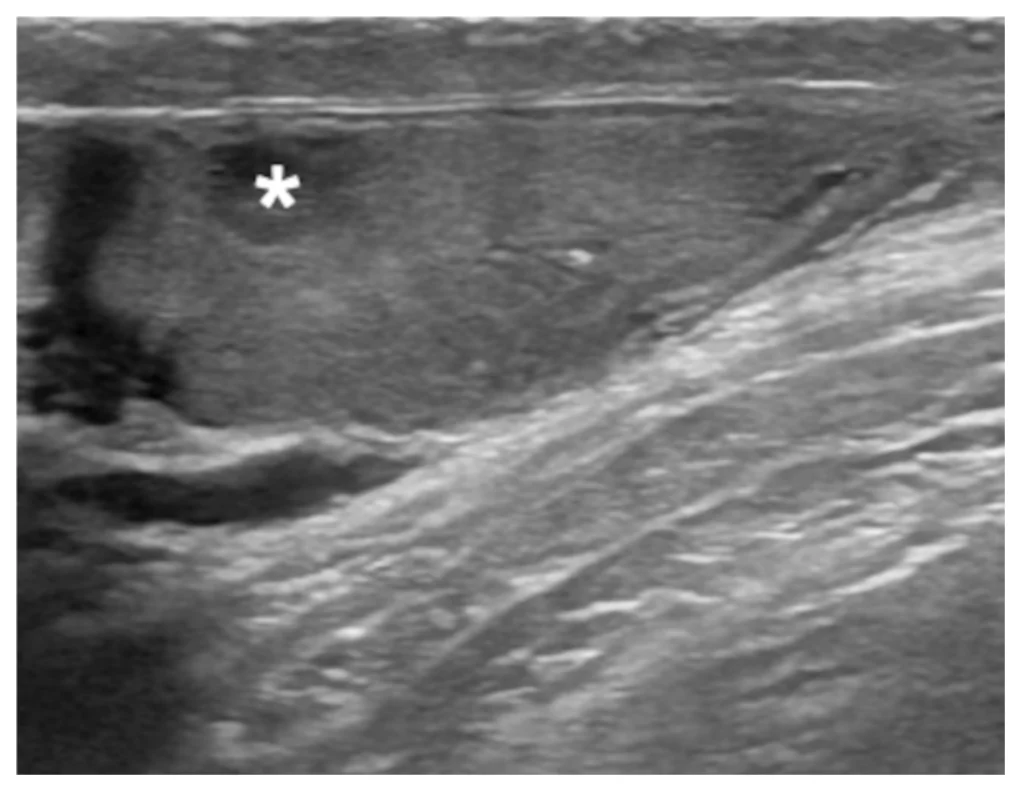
Small, non-palpable testicular lesion (asterisk) incidentally identified at ultrasound in a 45-year-old azoospermic man with bilateral testicular hypotrophy, investigated during fertility work-up. The lesion proved to be a seminoma at surgery.
